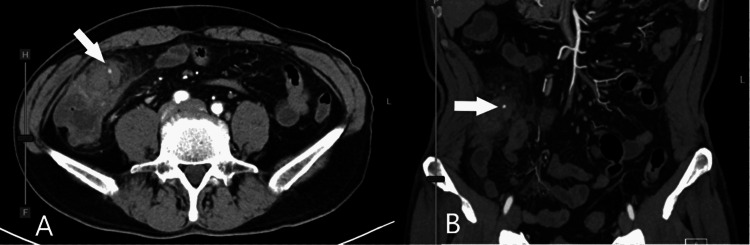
Abdominal contrast-enhanced CT scans in axial view (A) and coronal view (B) Pseudoaneurysm with hematoma was confirmed in the ileocolic artery (arrow).
